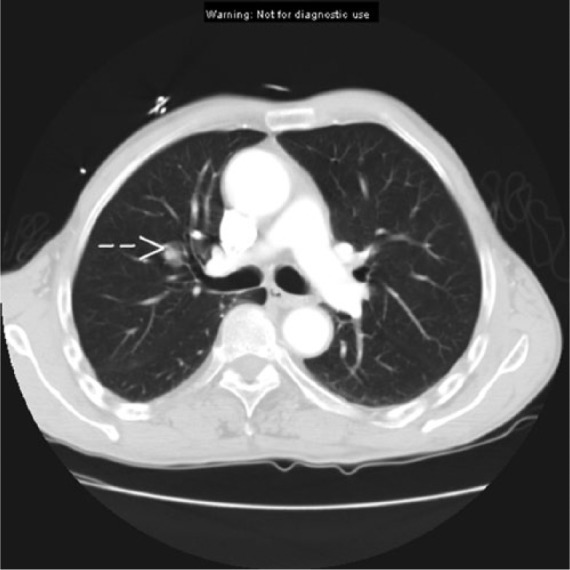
Computed tomography of the lungs demonstrating right pulmonary nodule.
